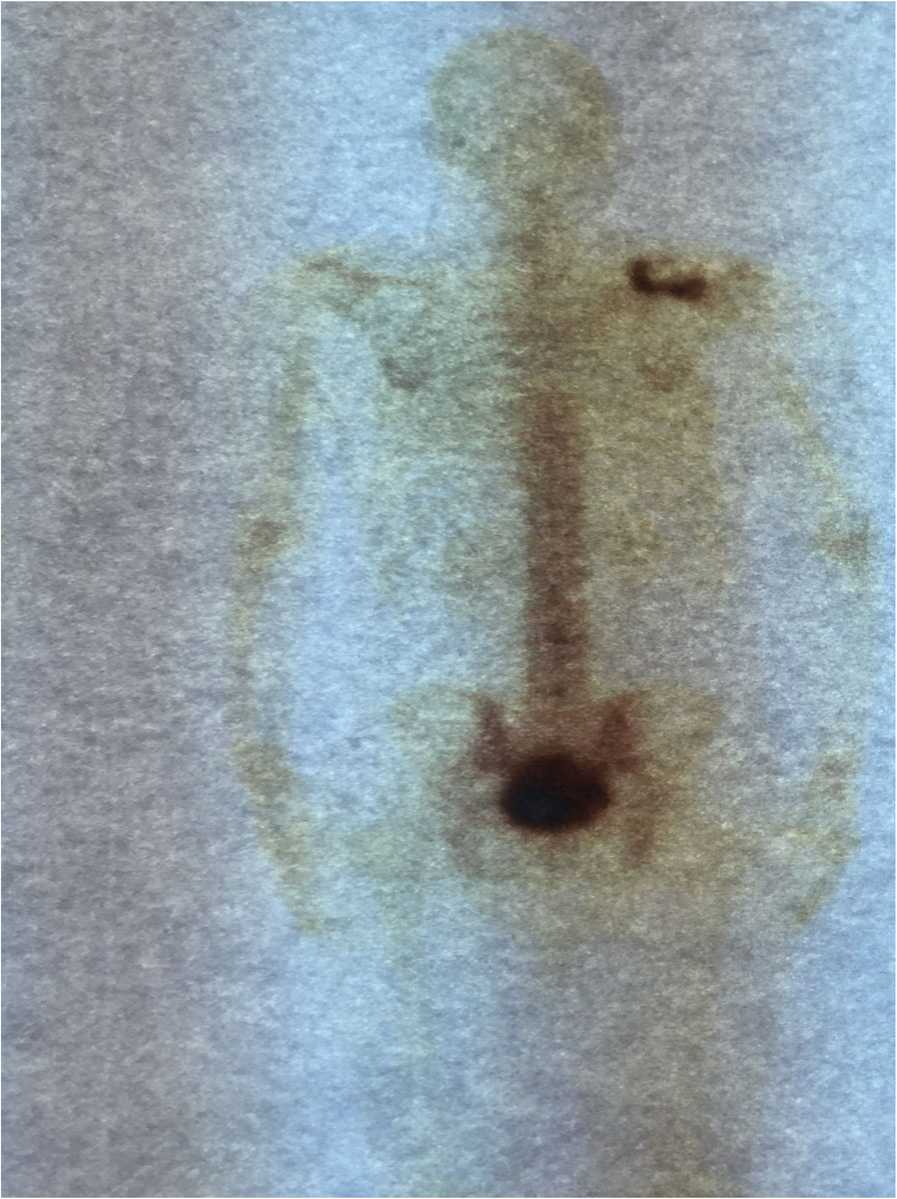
Bone scintigraphy with hyperfixation focus at the right scapula
